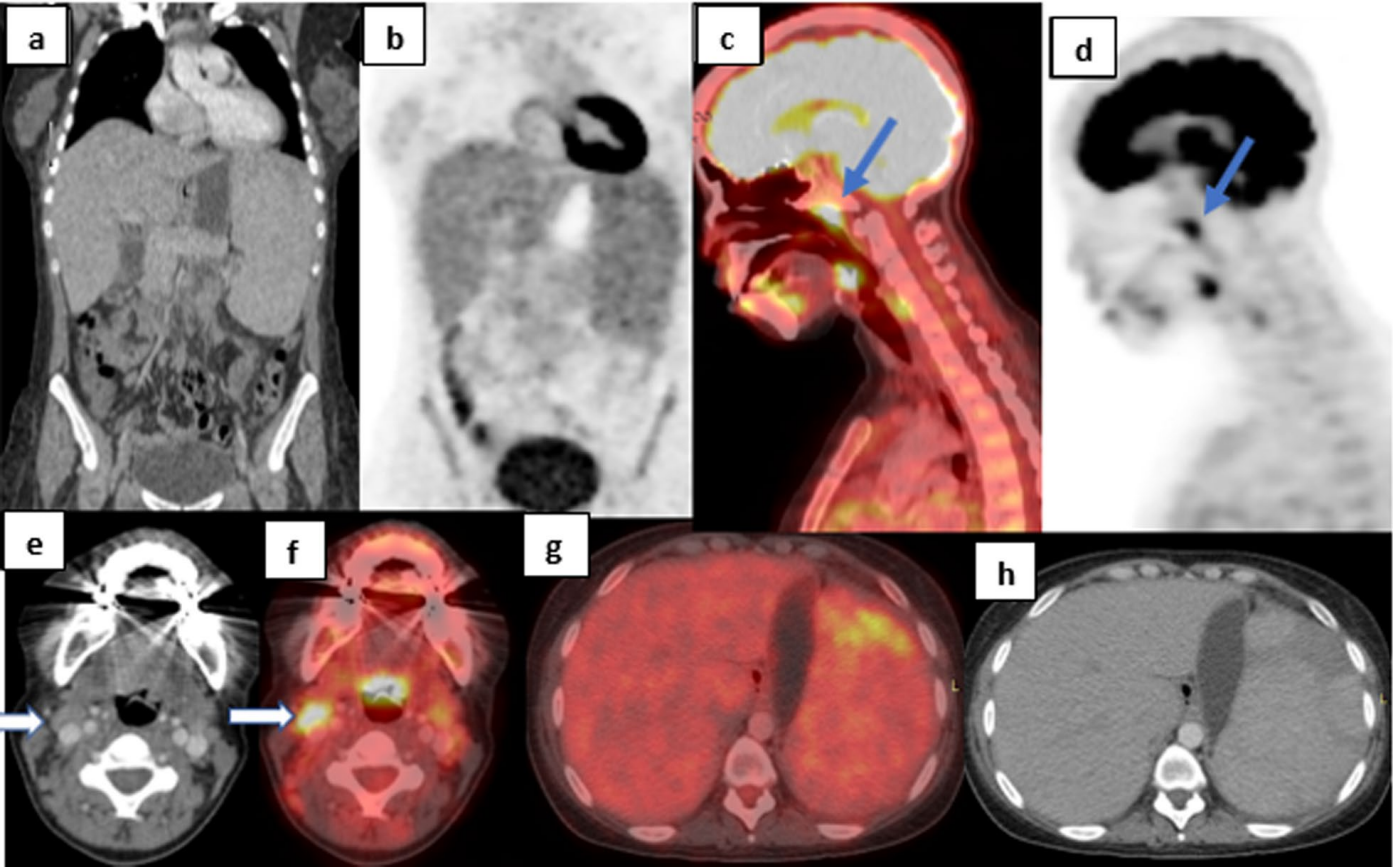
34-year-old female patient presented with fever of unknown etiology, fatigue and mild sore throat. a Coronal CT image, b Coronal PET image with attenuation correction showing enlarged liver and spleen with mildly increased splenic activity. c Sagittal PET/CT fused reconstructed image. d Sagittal PET attenuation corrected image, both showing increased nasopharyngeal activity with SUV max of (blue arrows). e Axial CT image of the neck. f Axial fused PET/CT image of the neck at the same level sowing right cervical metabolically active lymph nodes. g, h axial fused PET/CT and axial CT of the abdomen showing the enlarged spleen with ill-defined area of increased activity of its anterior pole. Other findings included diffuse increased marrow activity with SUV max of 3.3(not shown here). Her work-up showed positive Epstein Barr IgM
